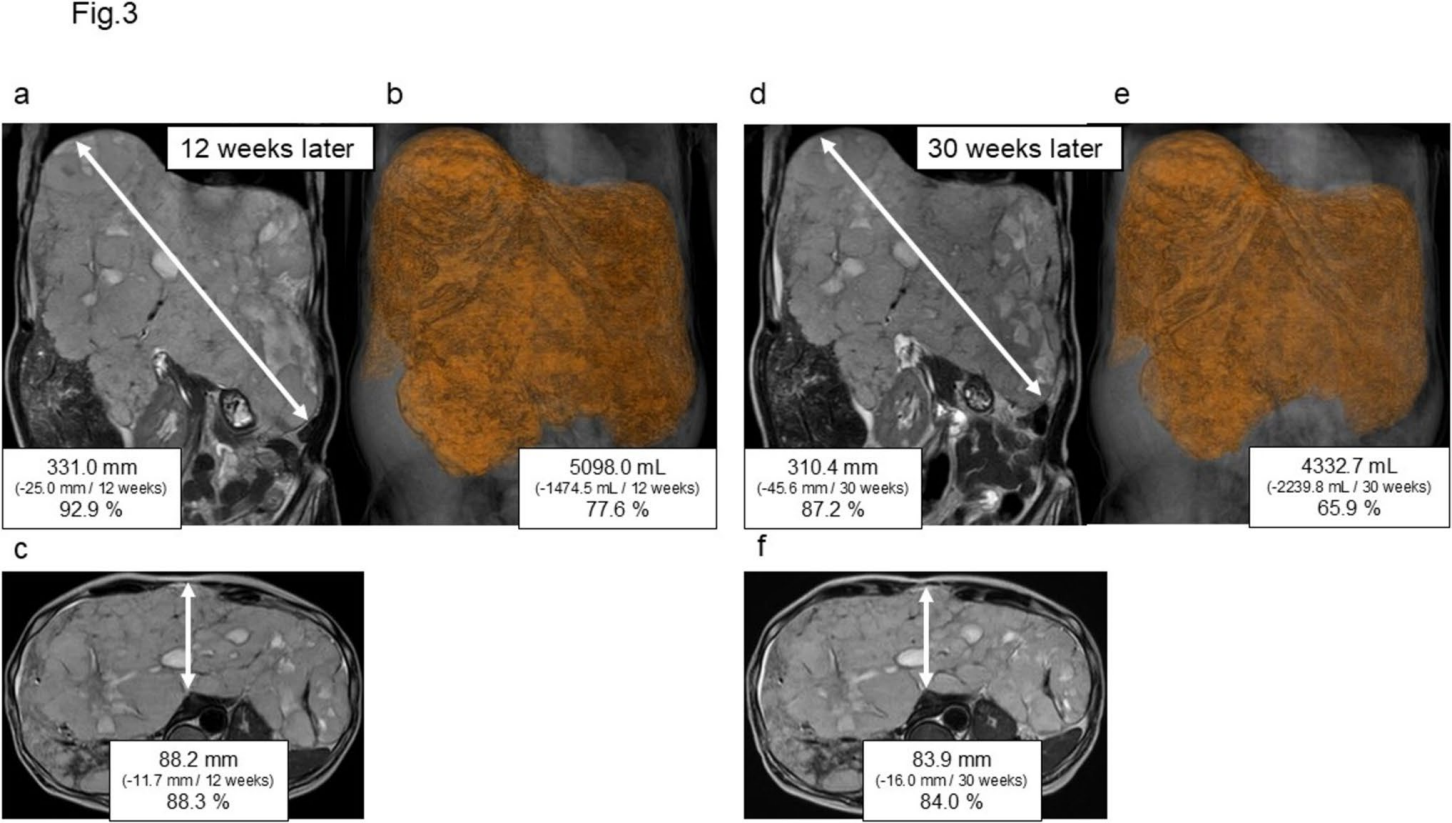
MRI of the giant hemangioma obtained at 12 (a–c) and 30 (d–f) weeks after initiation of sirolimus therapy. a The maximum diameter is 331.0 mm, representing a decrease of 25.0 mm from baseline. b The volume decreased to 5098.0 mL, a reduction of 1474.5 mL from baseline, corresponding to 77.6% of the initial volume. c The distance to the anterior abdominal midline is 88.2 mm. d The maximum diameter is 310.4 mm, representing a decrease of 45.6 mm from baseline. e The volume decreased to 4332.7 mL, a reduction of 2239.8 mL from baseline, corresponding to 65.9% of the initial volume. f The distance to the anterior abdominal midline is 83.9 mm, representing a decrease of 16.0 mm from baseline
